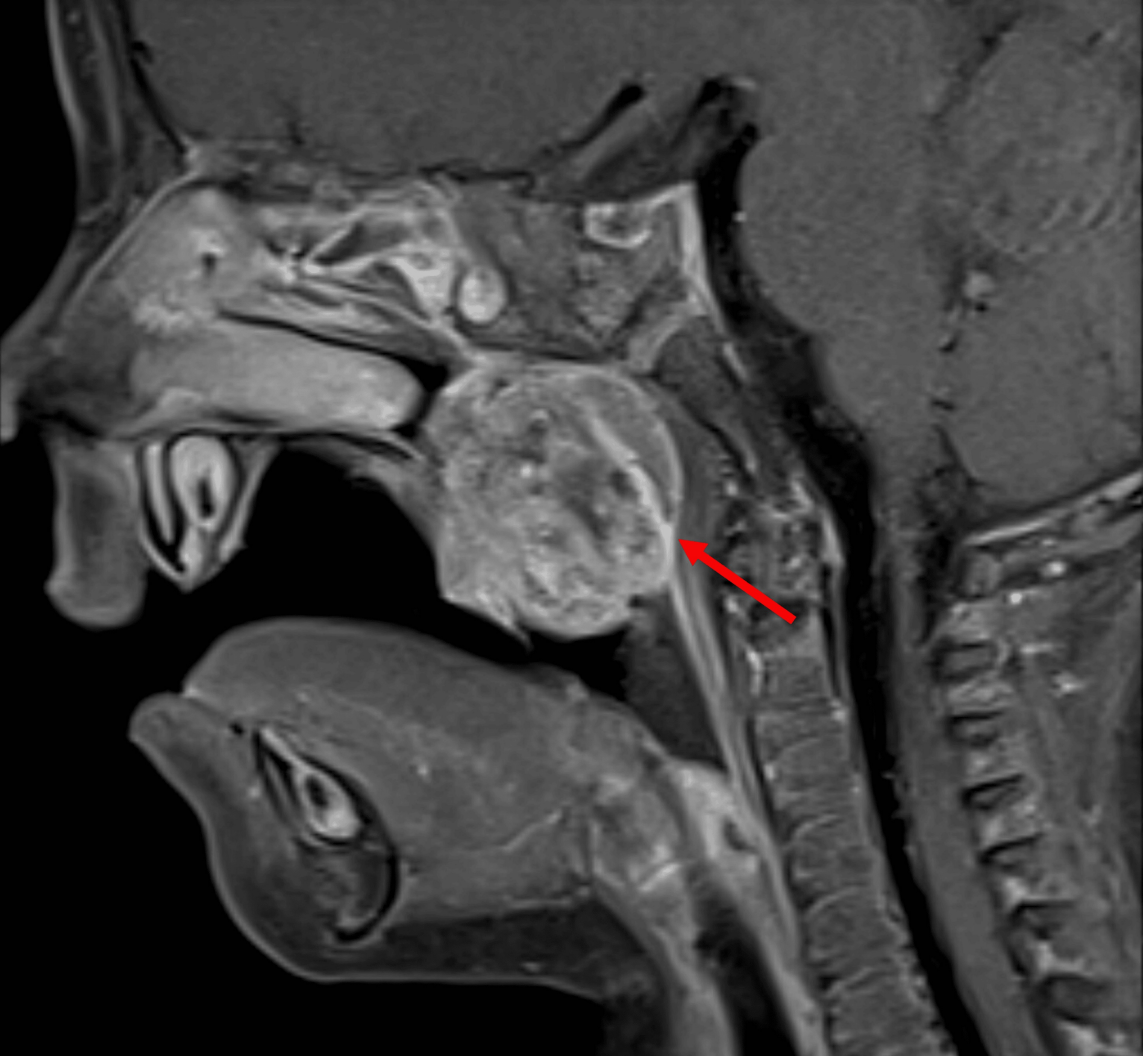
Case 1 MRI Sagittal MRI showing the mass, as highlighted by the red arrow, with a visible lack of communication to the intracranial space.
